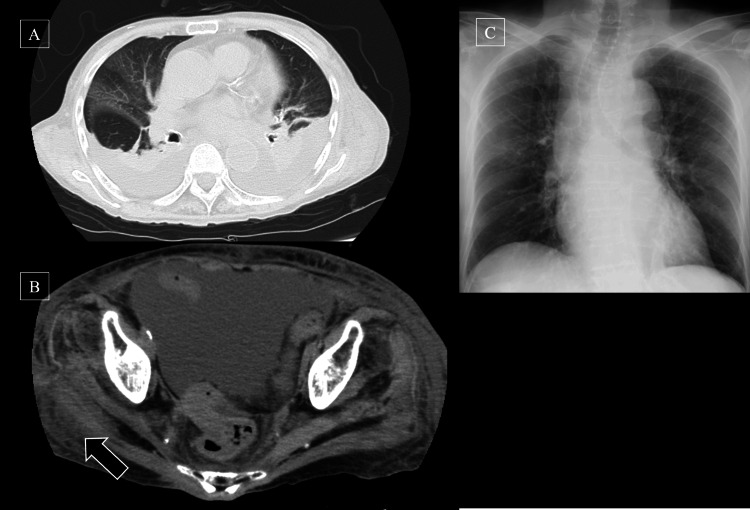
Radiological findings (A) Chest findings on plain computed tomography at the first admission: no apparent abnormalities were noted, except for ascites likely due to cirrhosis. (B) Leg findings on plain computed tomography at the first admission: swelling and fat stranding were observed in the right leg (arrow). (C) Chest X-ray at the second admission: no apparent abnormalities in the lungs or heart
